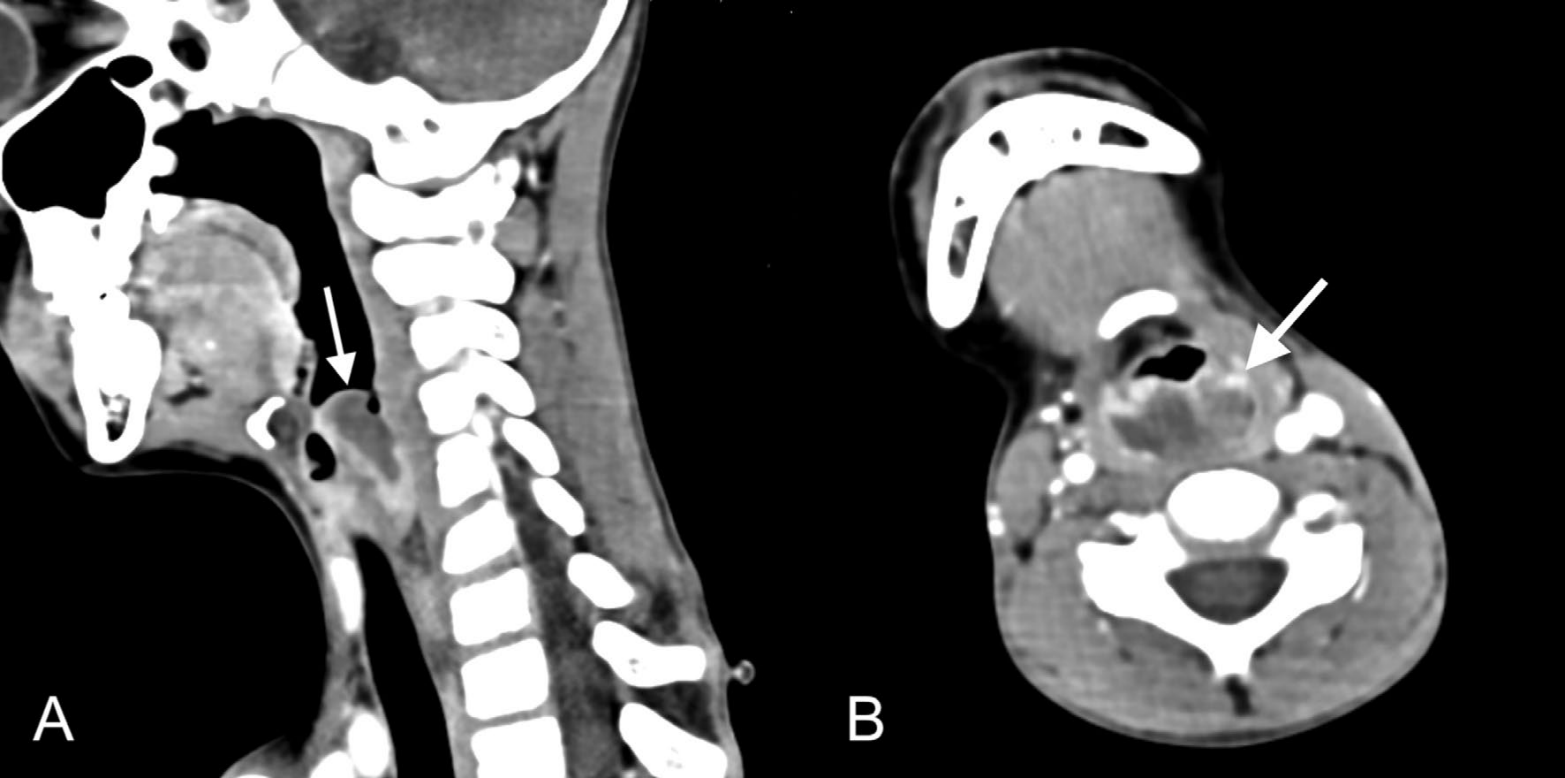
Contrast‐enhanced CT neck showing a supraglottic lesion, 8 months posttransplant. Sagittal (A) and axial (B) images show a heterogeneous lesion (arrows) with central hypoattenuation and peripheral irregular enhancement involving the aryepiglottic folds, with narrowing of the supraglottic airway. Biopsy of the lesion demonstrated monomorphic posttransplant lymphoproliferative disorder presenting as EBV‐positive diffuse large B‐cell lymphoma.
